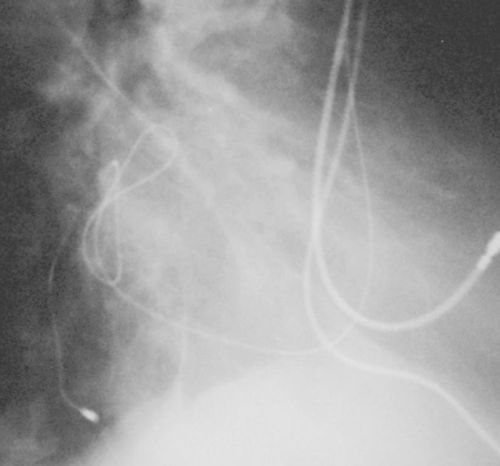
X-ray chest lateral view showing 'granny knot' of left ventricular lead in coronary sinus
